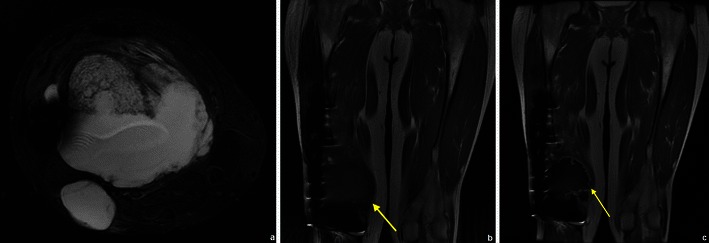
This patient had resection of the distal femur due to a chondrosarcoma. MRI showed a suspicious area of high signal near the distal prosthesis (a and arrow in b), which showed peripheral enhancing lobulations (arrow in c): a local recurrence of chondrosarcoma
